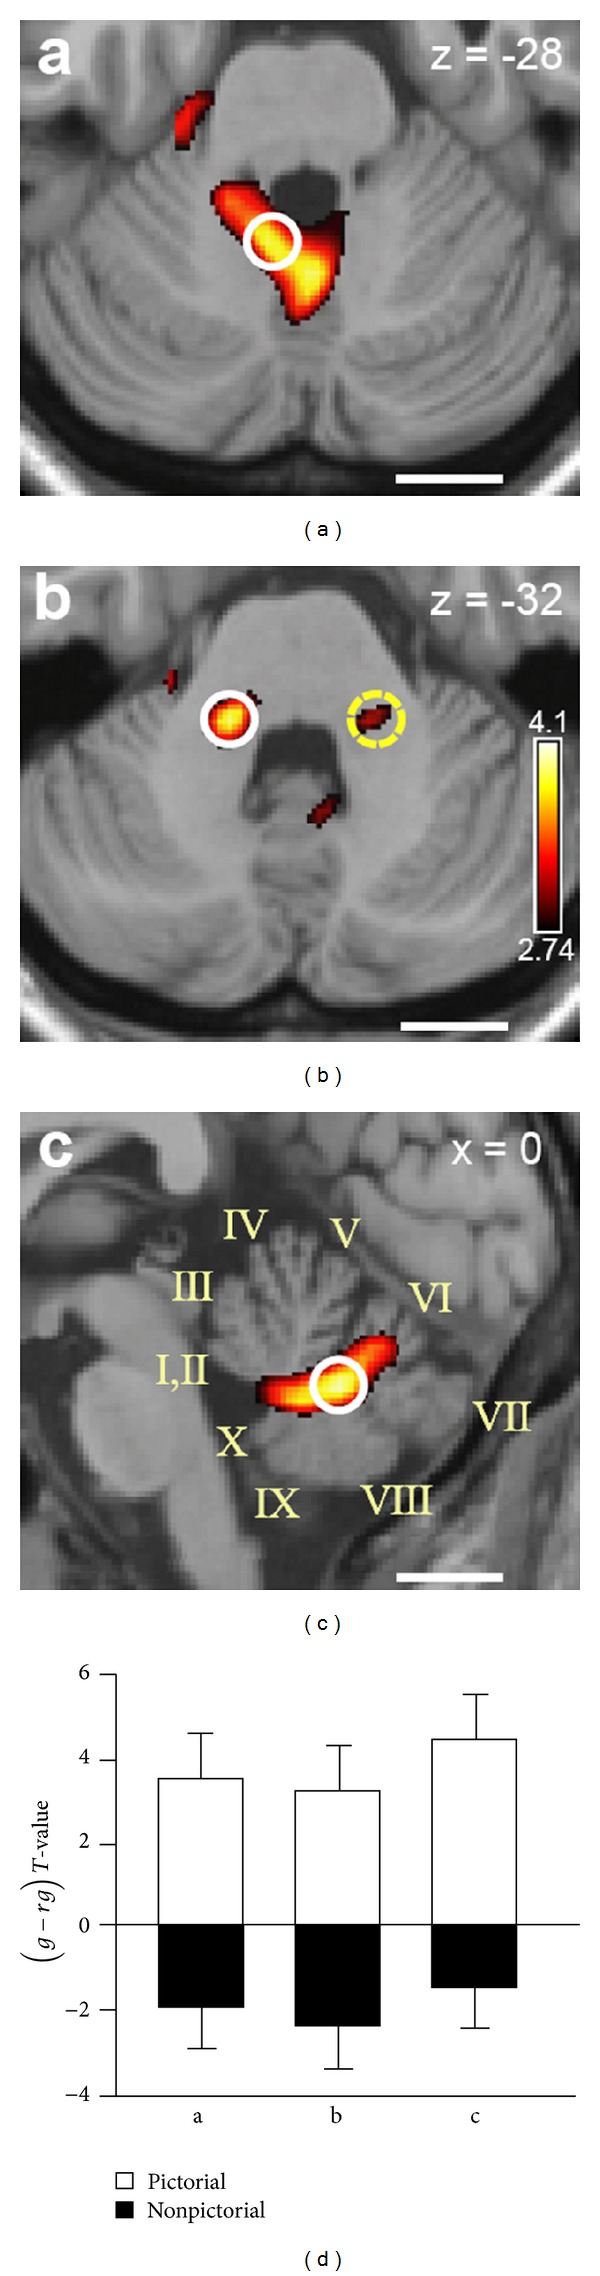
Functional magnetic resonance imaging of the cerebellum and brainstem in a task of interception of a ball moving along the vertical. Brain areas showing preferential activation for natural gravity motion specifically for the pictorial visual context. (a), (b), (c) Activations in the midline cerebellum ((a) axial section; (c) medial sagittal section) and vestibular nuclei ((b) axial section). Roman numerals in (c) denote Larsell lobules. White circles are centered on maximal statistical activation peaks. (a) Lobules IX/X. (b) Left vestibular nuclei. (c) Lobules VII/VIII. (d) Bar-graphs of the difference (± between-subjects s.e.m.)* t*-values for natural gravity (*g*) and artificial reversed gravity (*rg*) trials in pictorial (white) and nonpictorial (black) context for the activity peaks circled in (a), (b), and (c) (reproduced with permission from [7]).
